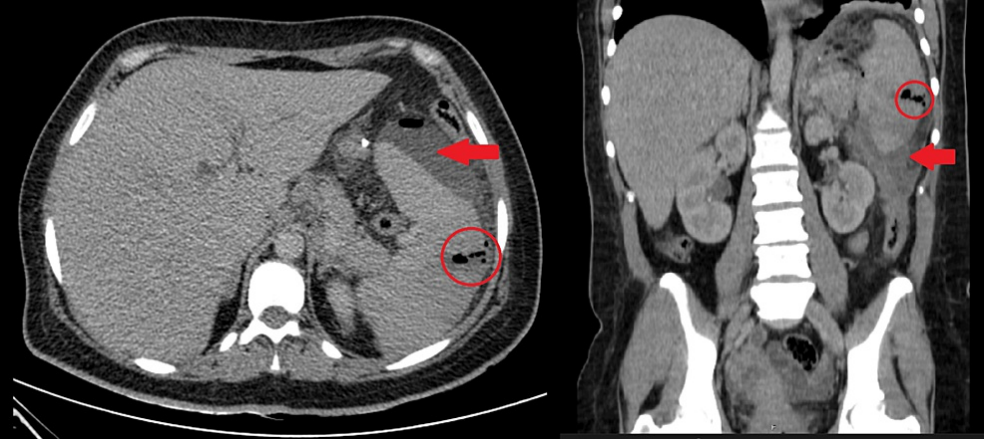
A CT scan upon admission showed a splenic abscess. The spleen abscess is marked with a circle, and the perisplenic free fluid is marked with arrows.
